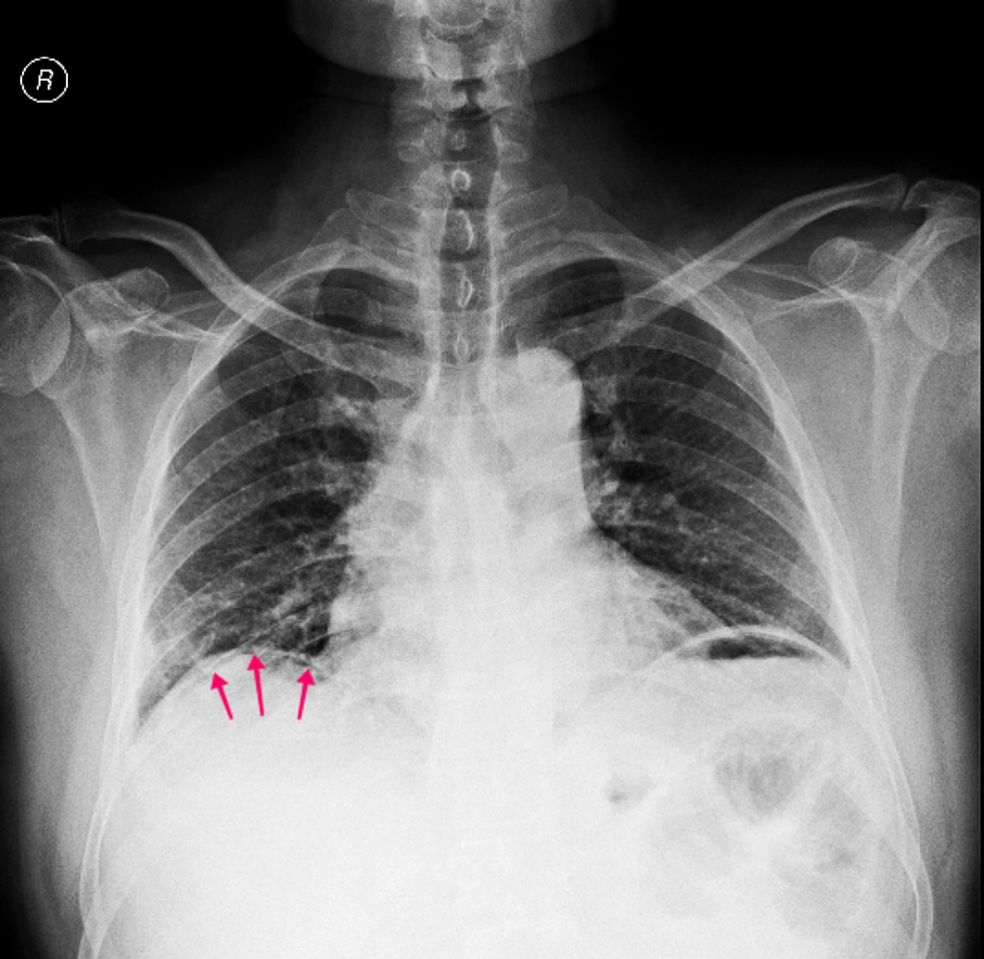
Chest X-ray showing a thin rim of air under the right diaphragm (red arrows).
